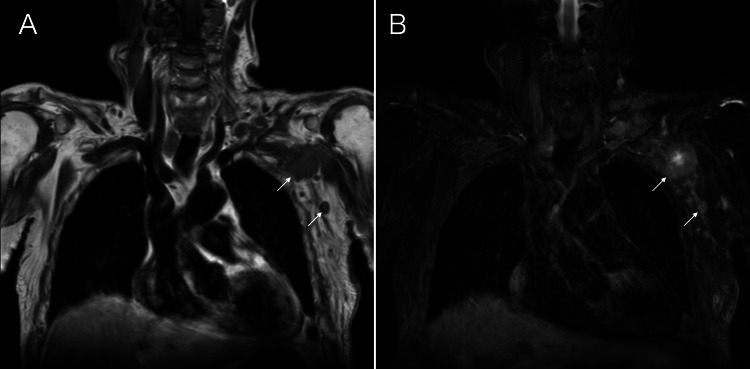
Magnetic resonance imaging (MRI) findings MRI of the axillary masses shows low signals on T1-weighted images (A, arrows) and predominantly very weak high signals on fat-suppressed T2-weighted images (B, arrows).
